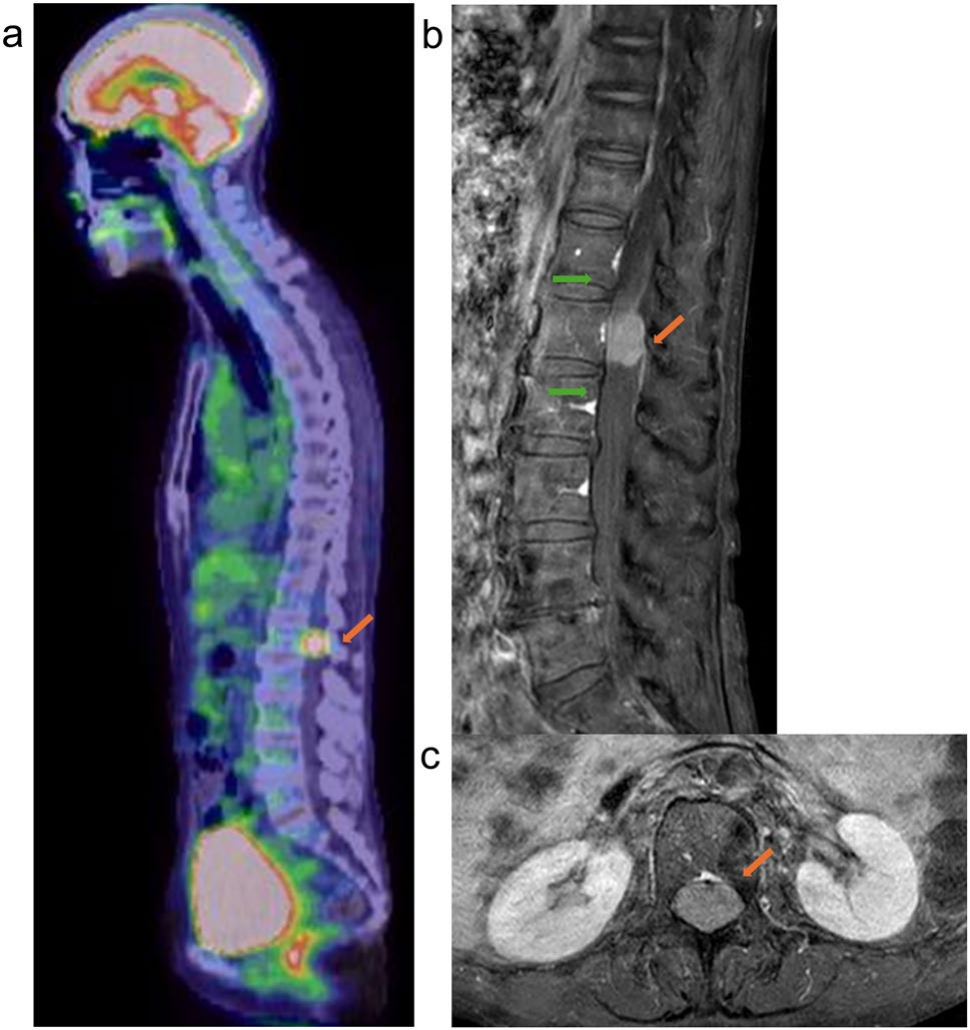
a FDG-PET/CT showed accumulation in the spinal cord cavity at L1 level (indicated by arrow in a; sagittal fused PET–CT scan). b and c Post-gadolinium T1-weighted MRI images showed a relatively uniform enhanced tumor in the spinal cord cavity at L1 level (indicated by orange arrows in b; sagittal MRI and c; axial MRI). The flame sign was observed (indicated by green arrows in b). *FDG-PET/CT* 18F-fluorodeoxyglucose positron emission tomography/computed tomography, *MRI* magnetic resonance image
